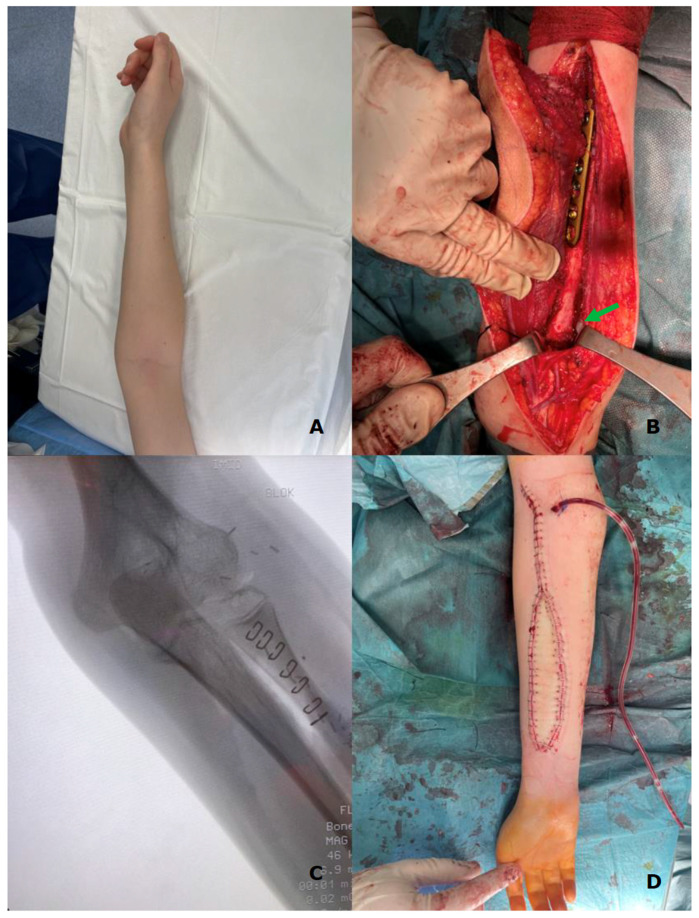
(A)—preoperative X-ray of the left upper limb with diagnosed Ewing Sarcoma of the radius; (B)—fibula free flap harvested with the fibular head (green arrow) placed within the wound bed after resection of the radial bone; (C)—intraoperative X-ray presenting the proper position of the fibular head within the annular ligament; and (D)—postoperative X-ray of the upper limb with a visible skin island of the fibula free flap (as a monitor of the appropriate perfusion of the buried part of the flap).
